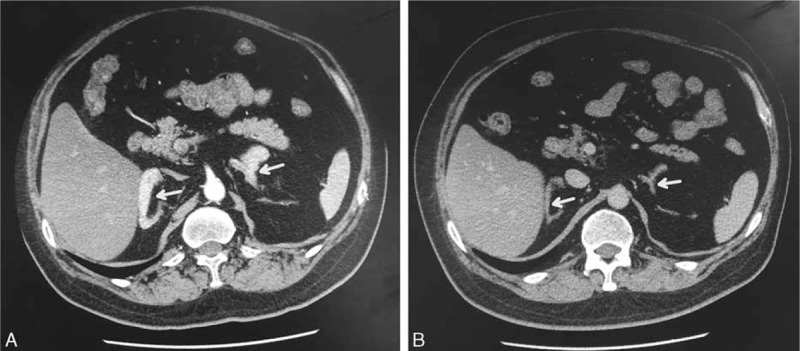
(A) Axial computed tomography (CT) images of the adrenals showing hyperplasia and enhancement of both glands before (white arrow) and (B) after the 4th surgical intervention. Both adrenal glands had almost returned to a normal size (white arrow).
